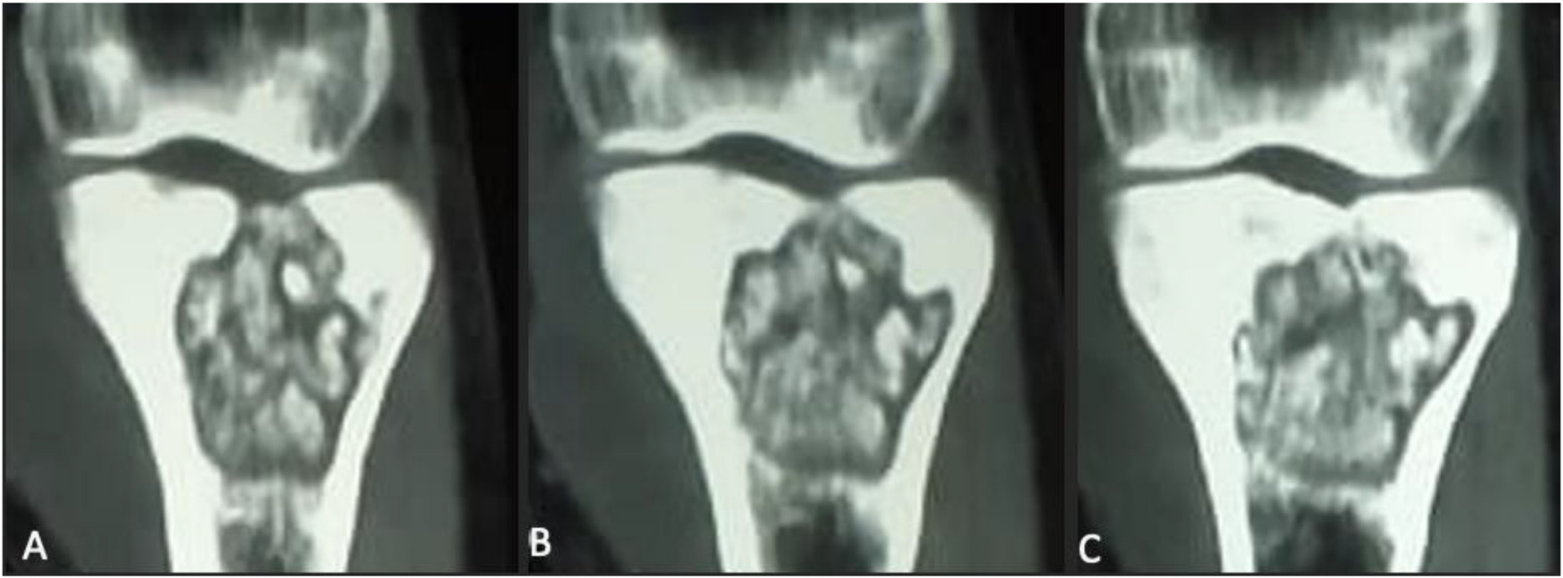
Coronal CT scans without contrast injection of the knee show a well-defined cavitary lytic lesion (C) in the metaphyseal-epiphyseal region of the tibia, with heterogeneous content, cortical bone lysis, and intra-articular extension into the knee joint (A and B).
